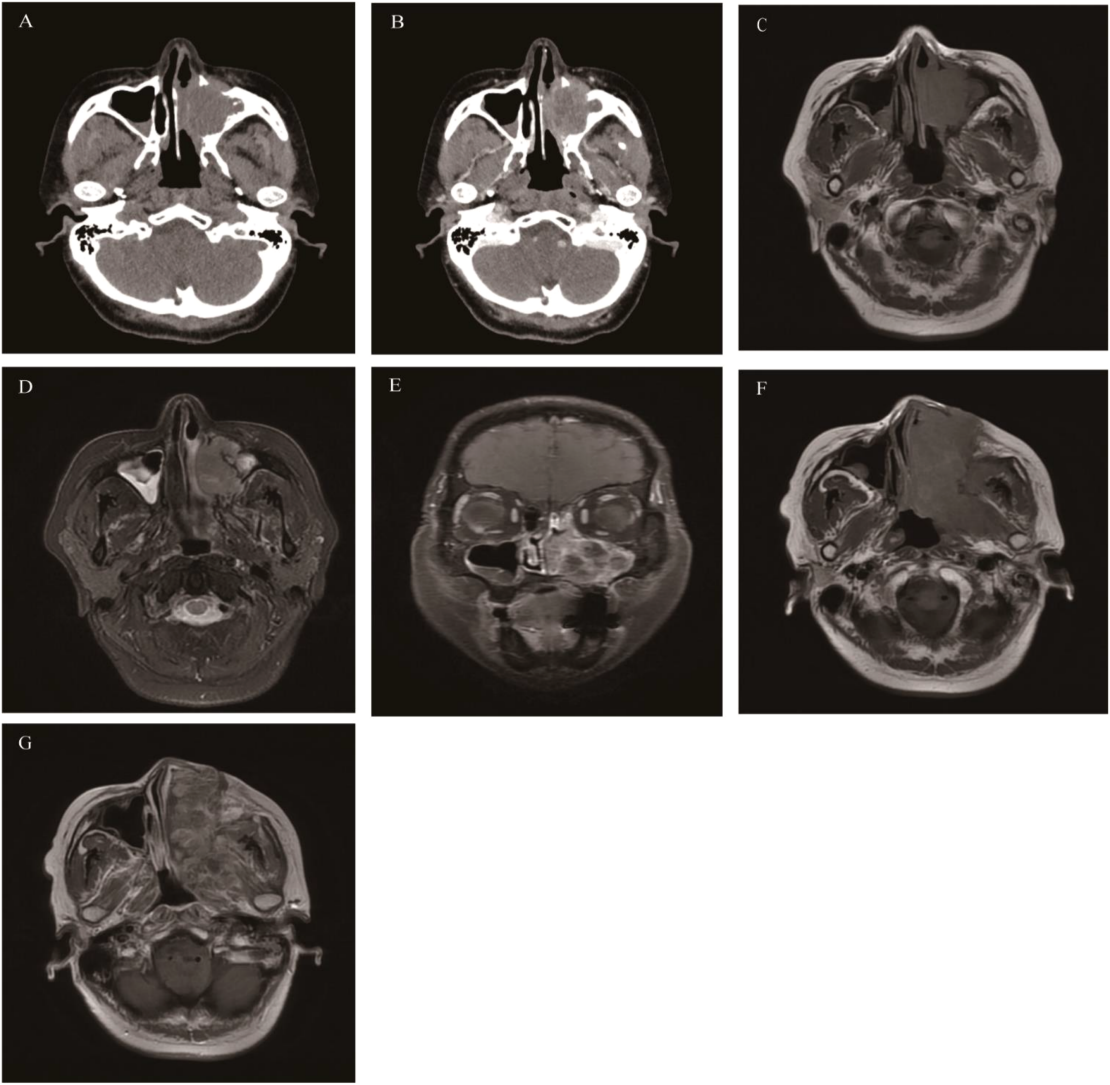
Squamous cell carcinoma: Axial CT showed an irregular soft tissue mass with bone destruction in the left maxillary sinus, exhibiting moderate inhomogeneous enhancement (A, B). T1WI showed an isointensity while T2WI indicated a slightly hyperintensity, both with moderate heterogeneous enhancement (C-E). Nine months later, the mass had significantly enlarged, extending anteriorly to the facial skin and posteriorly to the left parapharyngeal space on (F, G).
